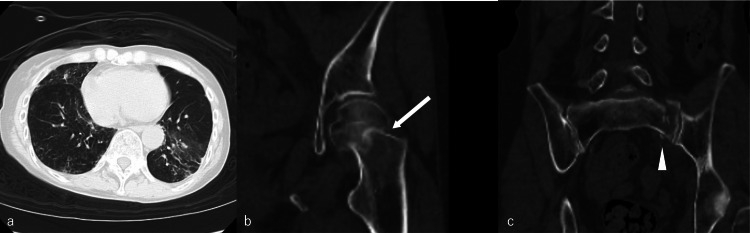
Thoracoabdominal and pelvic computed tomography at the time of the traumatic injury a) Preexisting interstitial pneumonia, b) Left femoral neck fracture (white arrow), c) Left sacral fracture (white arrowhead)
